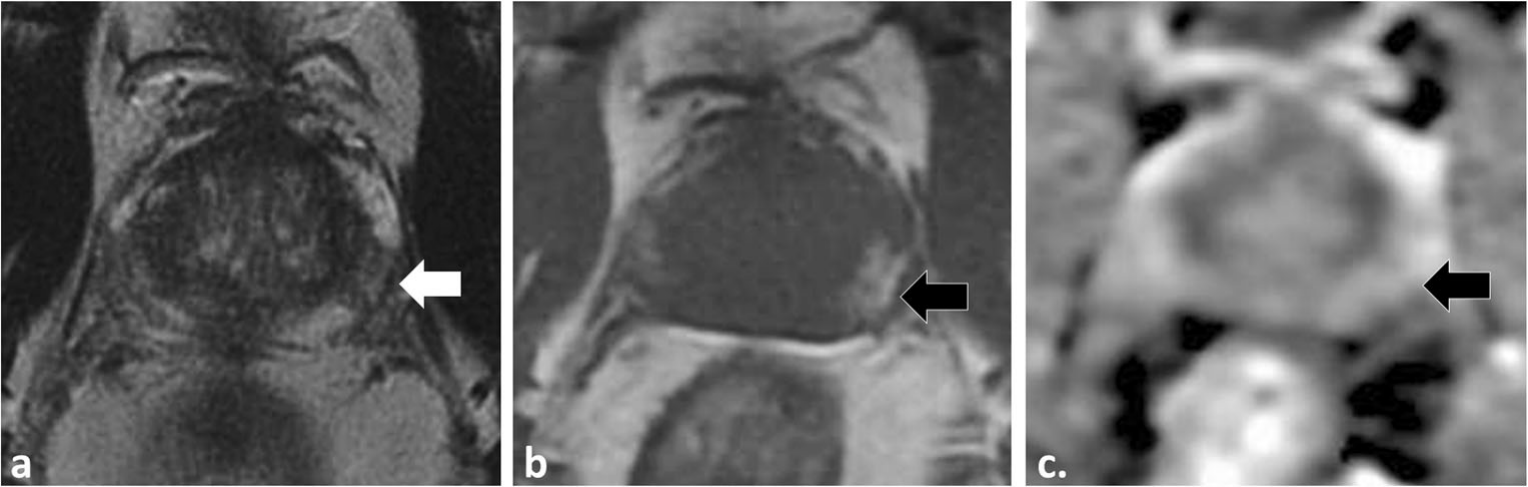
A 58-year-old male patient with Gleason score 3 + 3 = 6 tumour imaged with MP-MRI because of rising PSA. On axial T2W TSE (a) there is a low SI focus in the left mid PZ (*white arrow*), which could represent peripheral zone cancer. Corresponding axial T1W TSE (b) demonstrates increased T1 SI (*black arrow*) in keeping with post-biopsy haemorrhage. Axial ADC map (c) does not reveal the lesion (*black arrow*), which was readily diagnosed as post-biopsy haemorrhage
